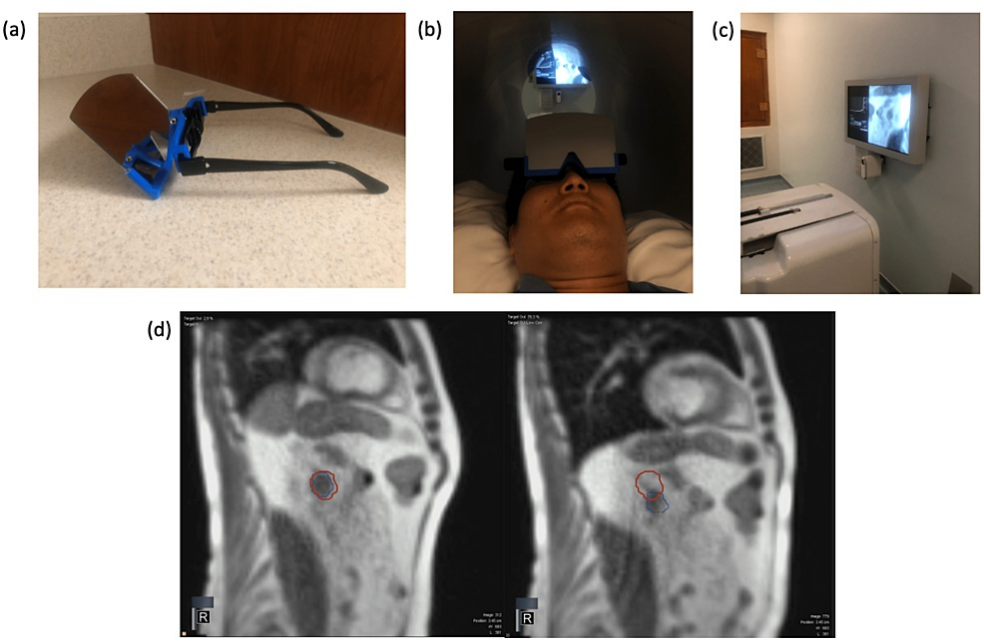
Magnetic resonance (MR) fraction delivery setup (a) Mirrored glasses used by the patient while lying in the bore of the MR-linac (b), allowing the patient to see the screen (c) with the tracking structure and boundary structure (d) target tracking structure on sagittal T1 MRI
